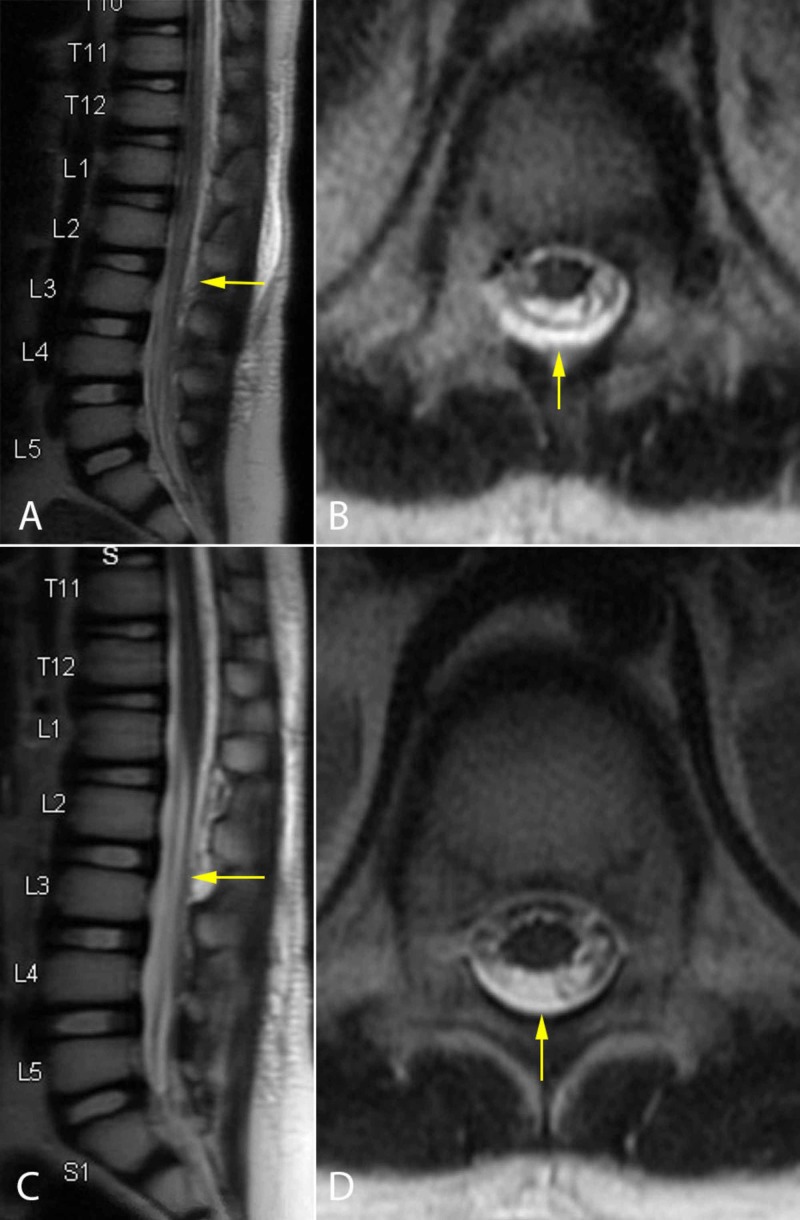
Case 1 Initial spine MR images showing the subcutaneous and epidural fluid collection (A and B). Postoperative spine MR images show resolution of the epidural fluid collection (C and D)
